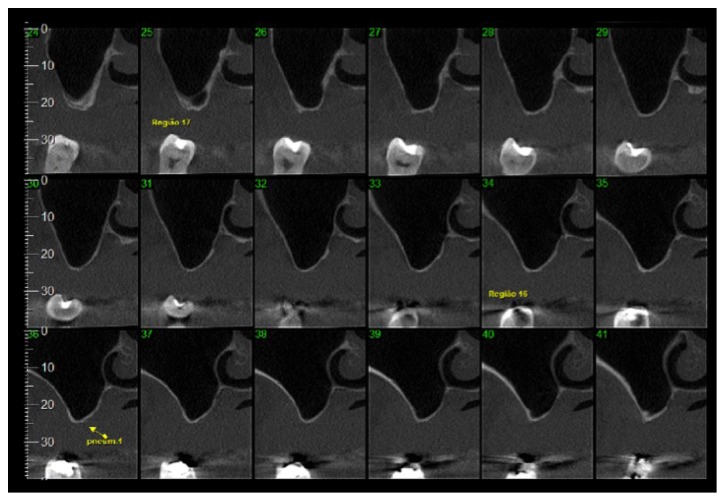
Cone-beam computed tomography scans of the posterior maxillary region.
